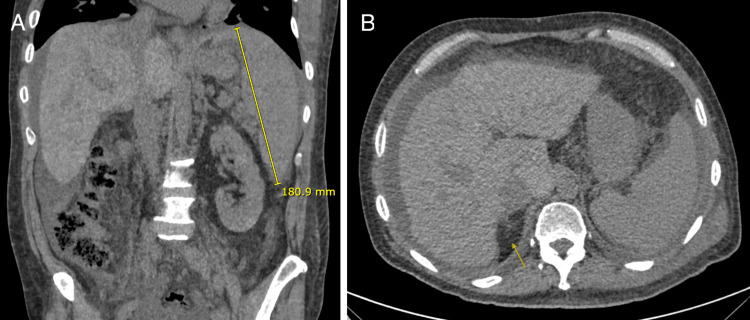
Abdominal CT scan (A) Coronal abdominal CT image demonstrating splenomegaly, with a maximal craniocaudal splenic length of 180.9 mm (normal adult splenic length ≤120–130 mm), consistent with clinically significant portal hypertension. (B) Axial abdominal CT image showing morphological features of chronic liver disease, including right hepatic lobe atrophy with a characteristic notch sign (arrow), defined as an indentation along the right hepatic contour reflecting segmental volume loss. Relative hypertrophy of the left lateral segment and caudate lobe, as well as a nodular hepatic contour, is also evident. Associated perihepatic and perisplenic ascites further support the diagnosis of portal hypertension.
